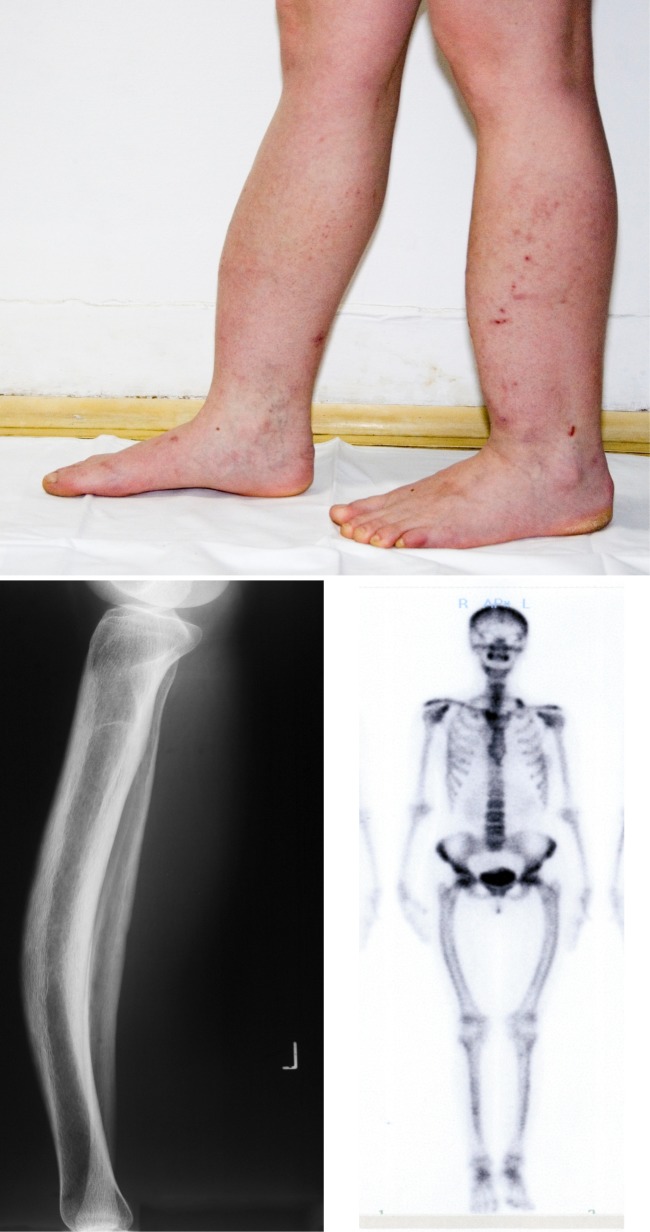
Counterclockwise from the top: Photograph showing deformity of the left and right tibia. Radiograph of the left tibia showing osteosclerosis and osteolysis. Radionuclide bone scan showing increased tracer uptake in both femur and tibia.
